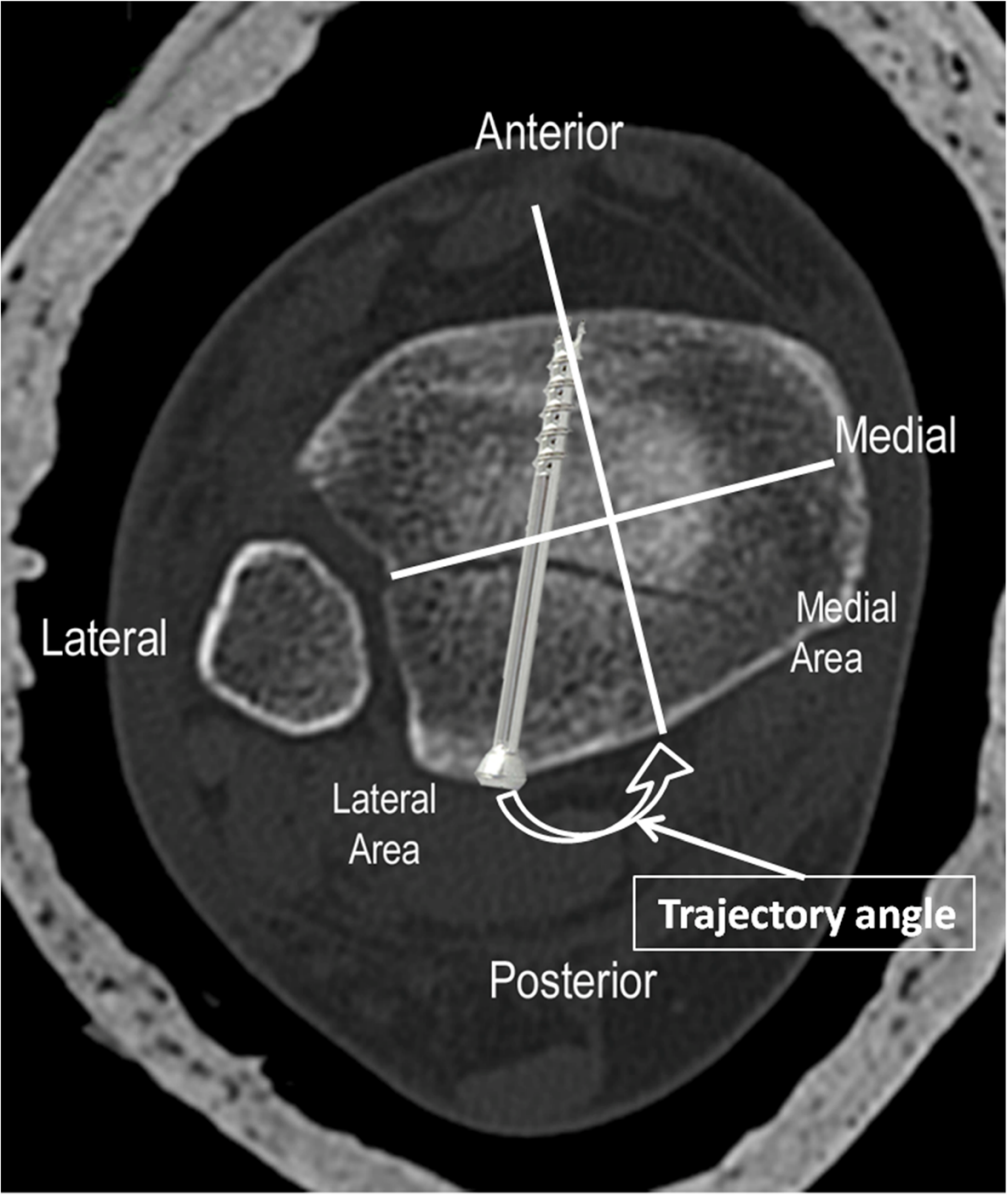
Axial CT image demonstrating the trajectory angle and ideal screw lag placement in postero-lateral type posterior malleolar fracture
